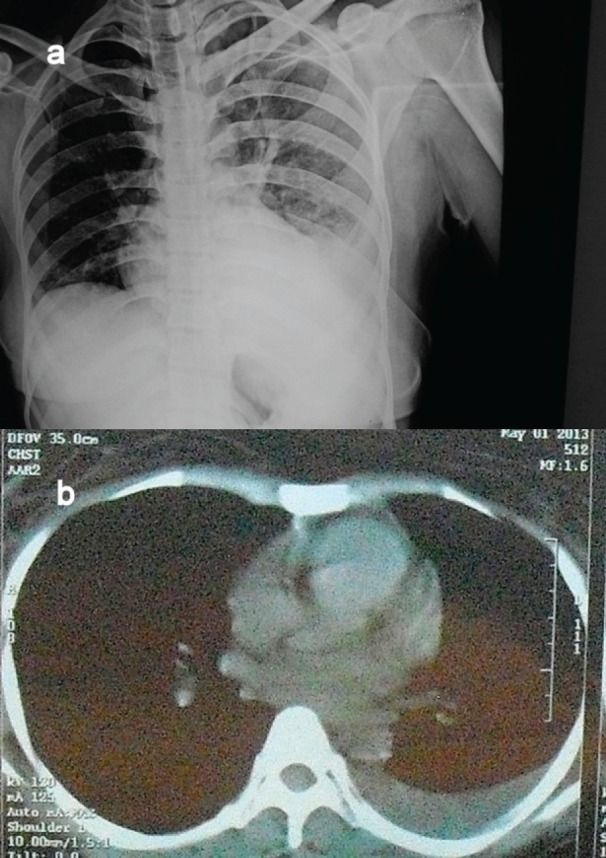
(a) and (b) Chest skiagram and CT scan of the patient, revealing left pleural effusion.
